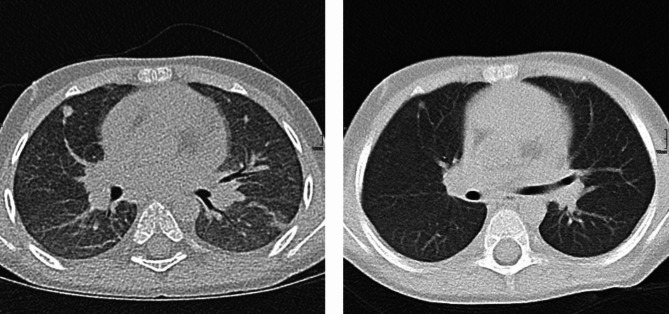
CT thorax before (left, September 2021) and after (right, November 2021) therapy initiation with prednisolone, intravenous immunoglobulins, cotrimoxazole and ruxolitinib. Left: pleural round spot between middle lobe and upper lobe; ventral milk glass opacities. Right: Remission of milk glass opacity in all lobes of the lung with single patchy residuals in the middle lobe and basal upper lobe on the right
